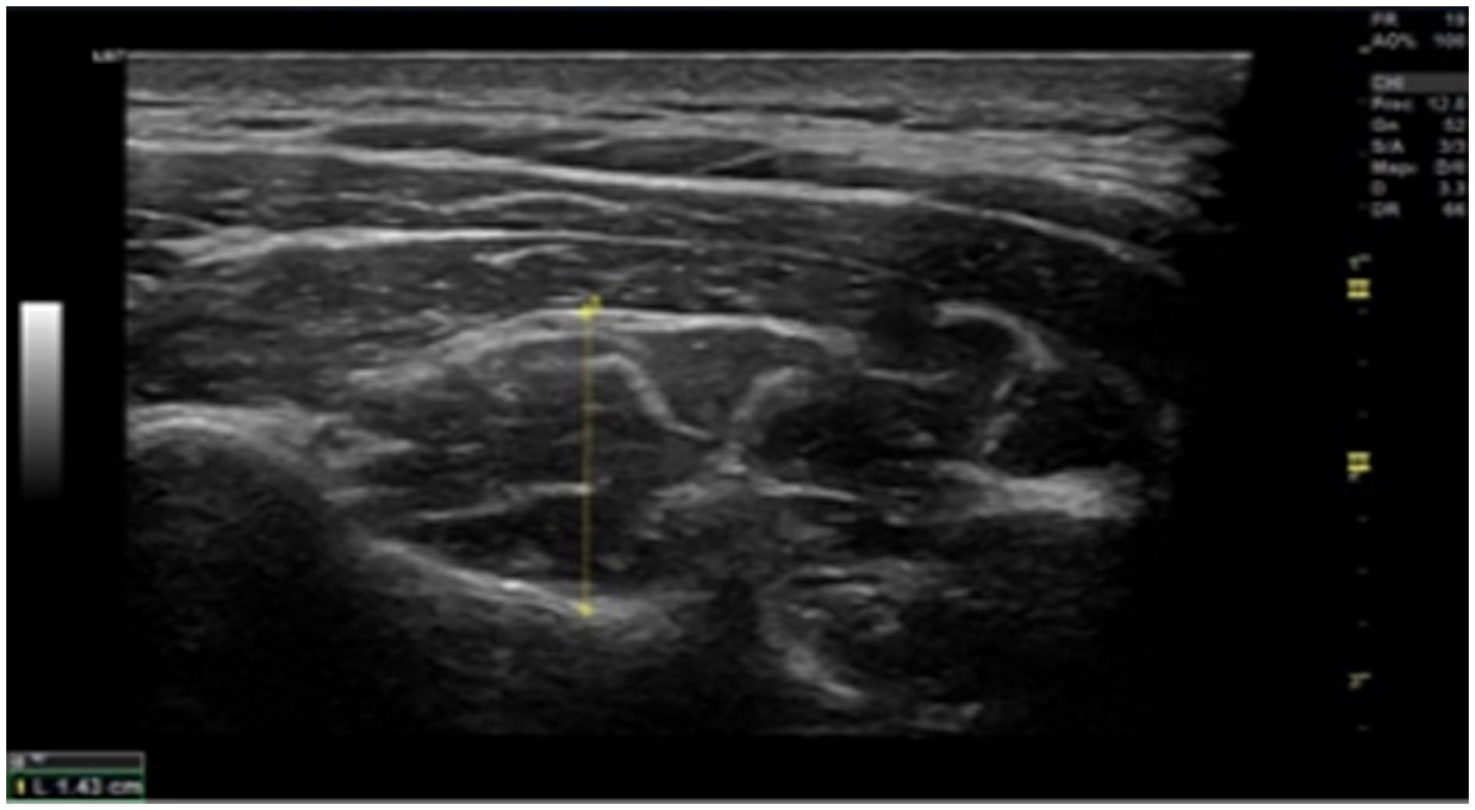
Cross-sectional image of a multifidus ultrasonography at C5. Up to the echogenic line of the hyperechoic fascia between the semispinalis cervicis and semispinalis capitis, the rater’s assessment of the muscle’s thickest point is where the probe is positioned 90° to the lamina of C5.
